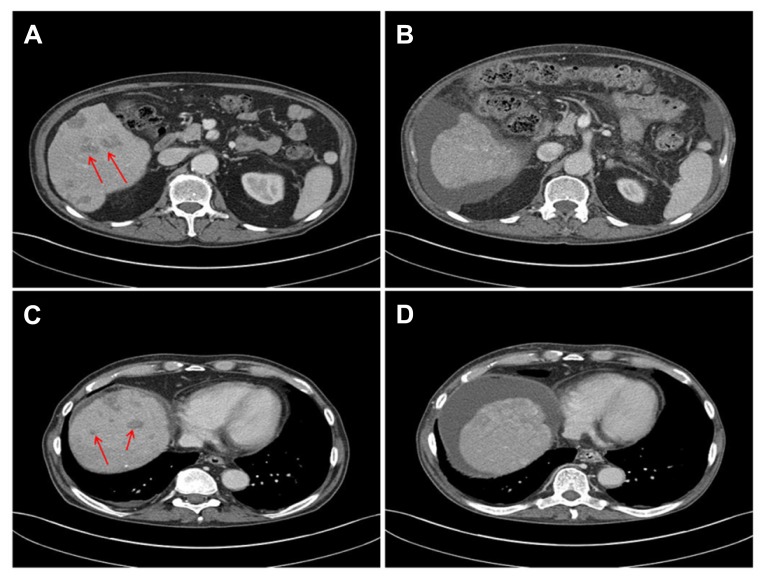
CT scans before (A,C) and 5 weeks after initiation of treatment with pemetrexed (B,D) show decrease in size of metastases and liver sclerosis and ascites due to previous embolization.
